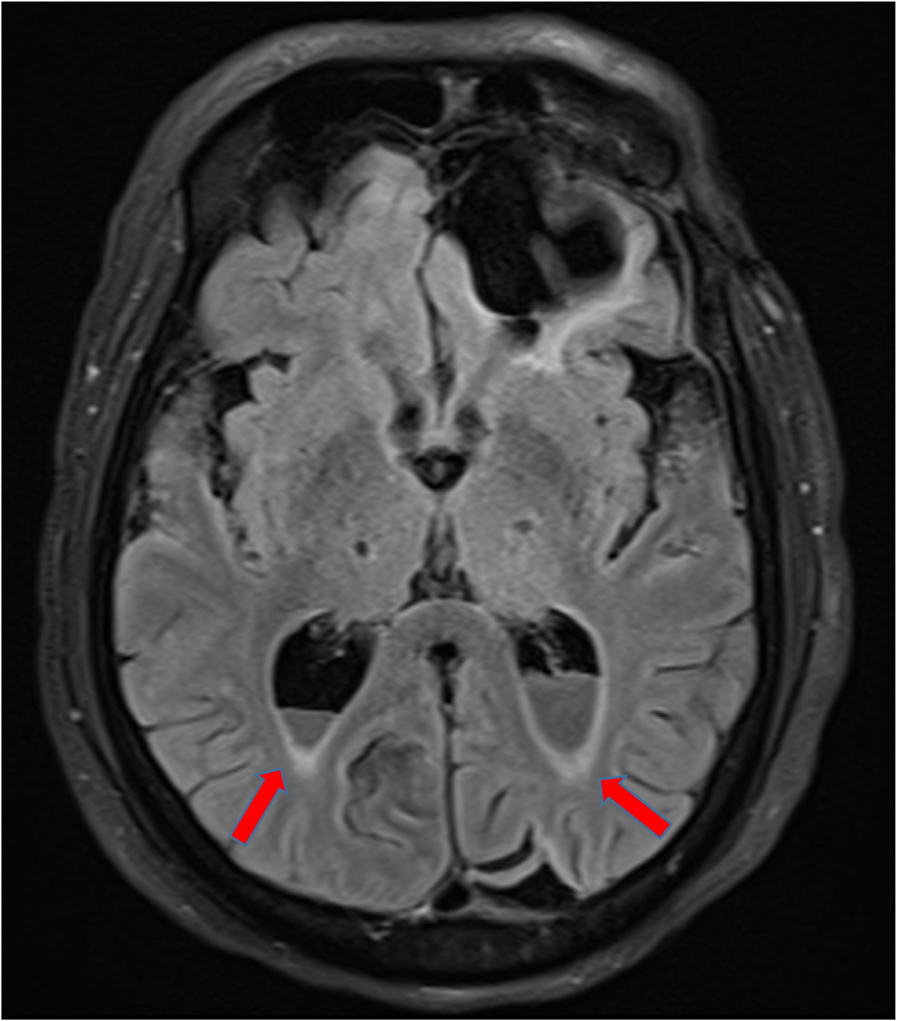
Post-gadolinium T2 FLAIR showing subtle ventricular ependymal enhancement and exudates (arrow) in occipital horns
